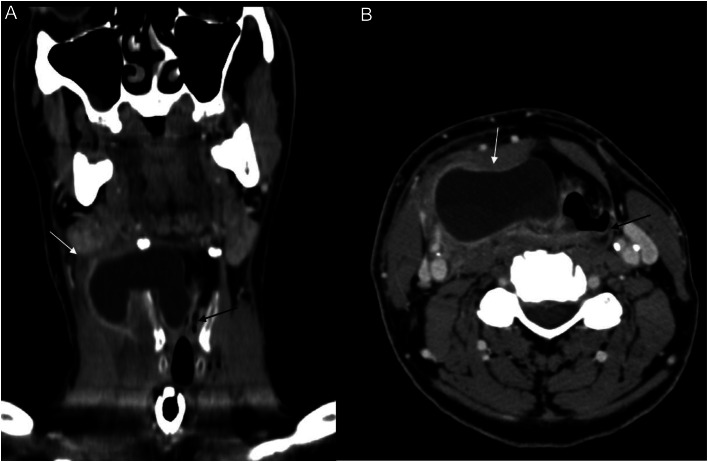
Coronal (A) and axial (B) contrast-enhanced computed tomography of the neck demonstrates a peripherally enhancing fluid collection arising from the right laryngeal vestibule, extending into the right anterior neck through the thyrohyoid membrane (*white arrow*), with narrowing of the laryngeal airway (*black arrow*), consistent with pyolaryngocele. Mild inflammatory changes noted in the surrounding soft tissues.
